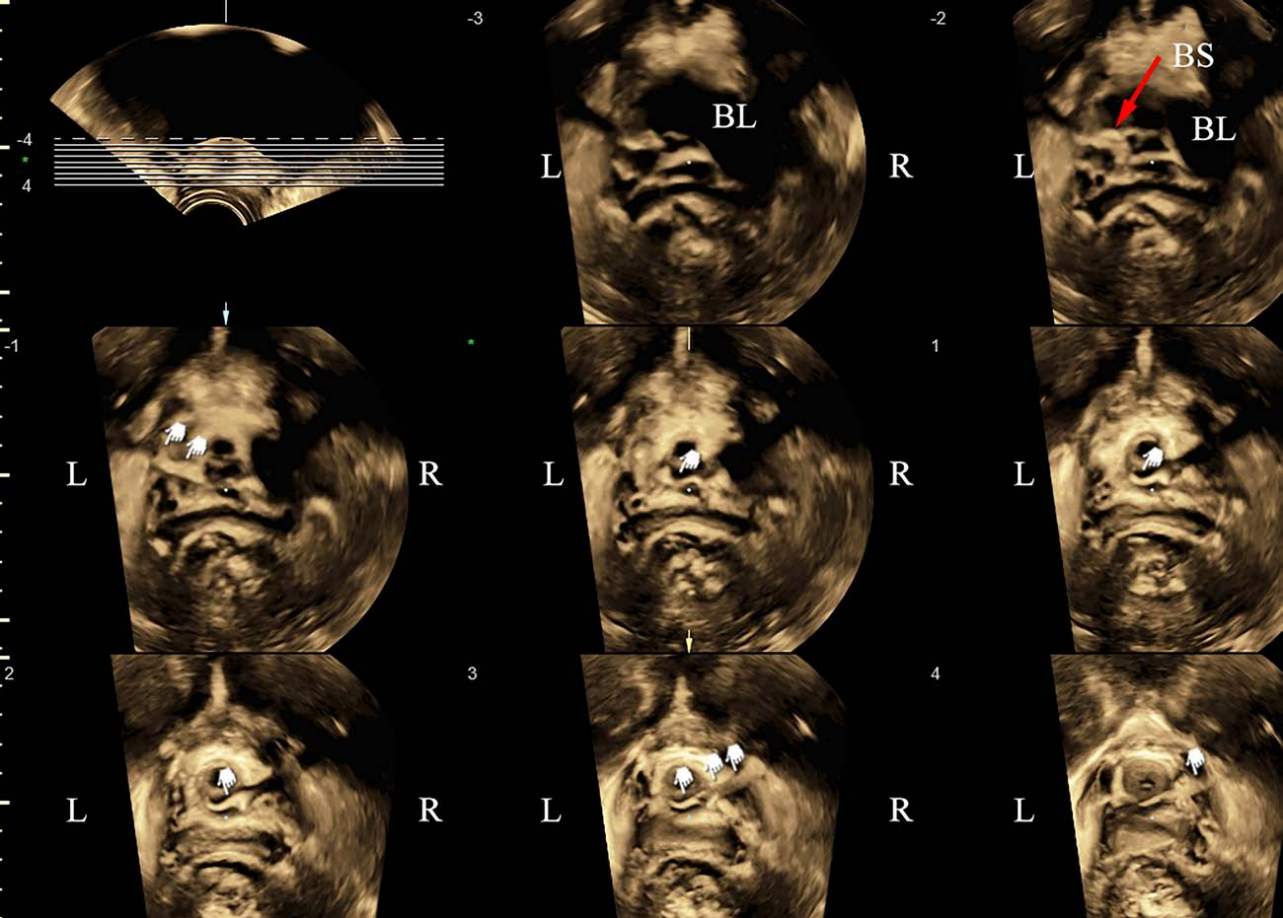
Three-dimensional (3D) ultrasound shows 8 tomographic images. The red arrow indicates the bladder stones. The white hand shapes show the track of sling. BL = bladder, BS = bladder stones, L = left, R = right.
